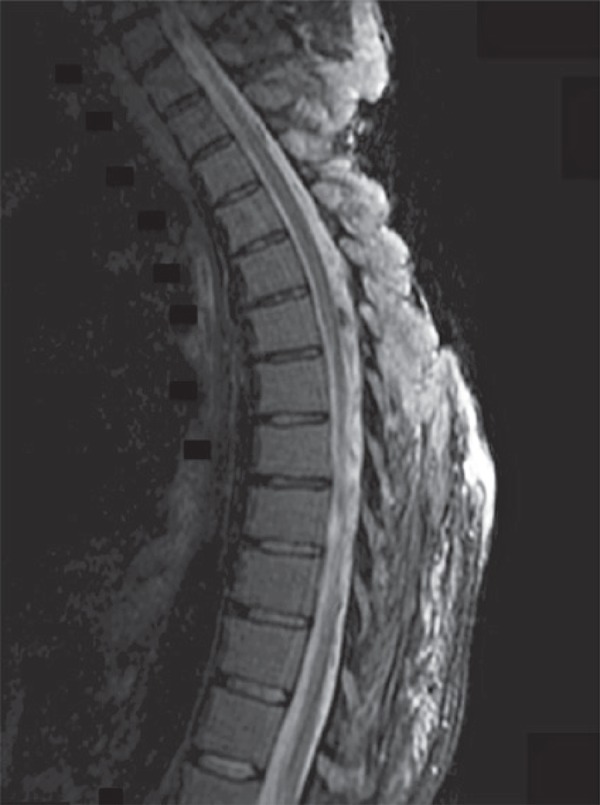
Midline sagittal view of the spine using magnetic resonance imaging demonstrating absence of spinal cord involvement
